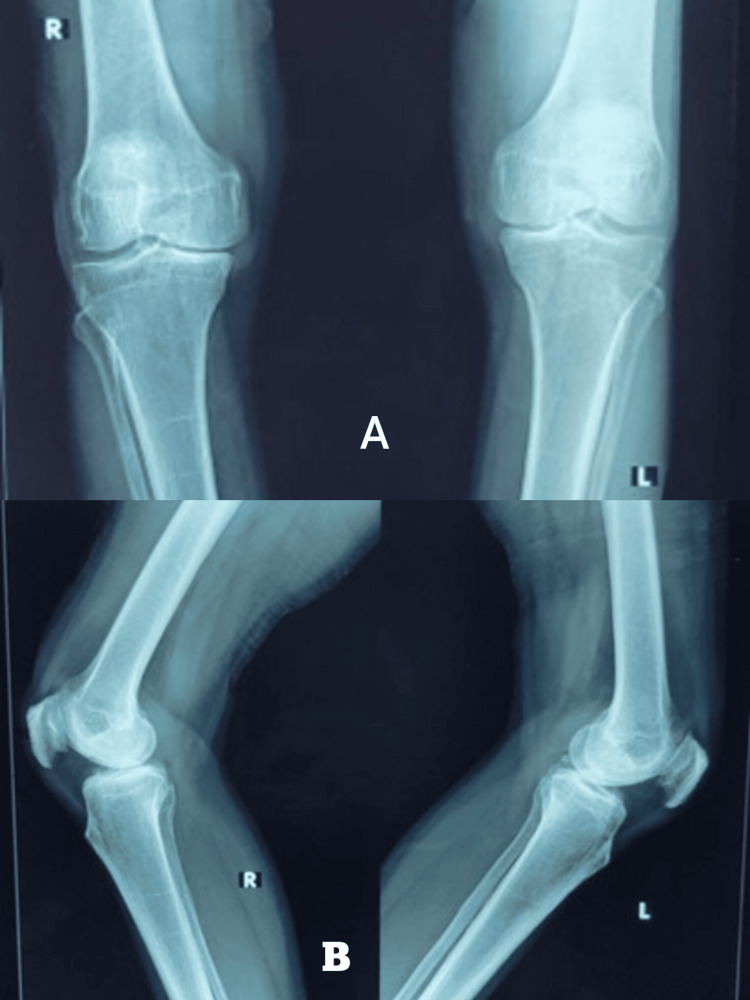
Preoperative radiographs of bilateral knee of a participant (A) Anteroposterior view; (B) Lateral view
